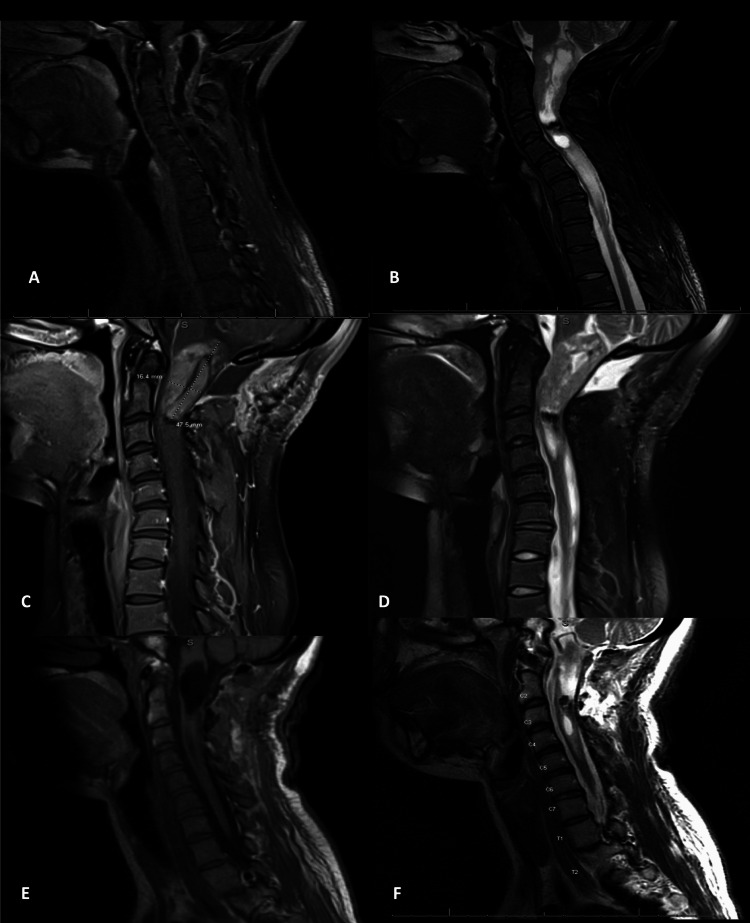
Sagittal magnetic resonance imaging findings. (A) Preoperative T1 image with contrast. (B) Preoperative T2 image. (C) Post-biopsy and drainage T1 image with contrast. (D) Post-biopsy and drainage T2 image. (E) Post-resection T1 image with contrast. (F) Post-resection T2 image.
